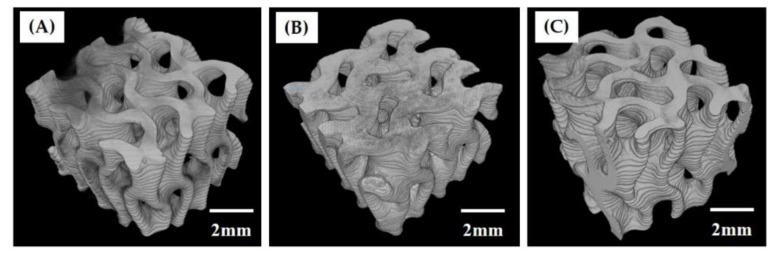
Representative micro-computed tomography (μ-CT) images of the hierarchically porous CaP scaffolds manufactured using a range of camphene-camphor amounts: (A) 40 vol %, (B) 50 vol %, and (C) 60 vol %.
